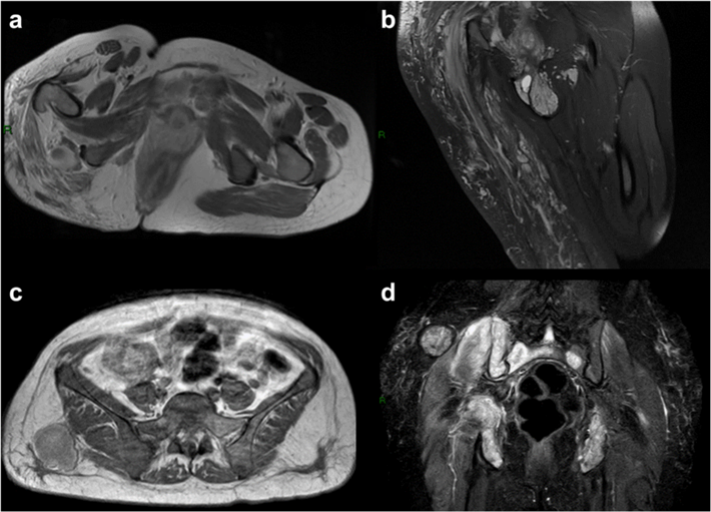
Traditional MR imaging. Axial T1 with contrast media and coronal DP fat of huge angiomatosis of the gluteal region and upper right tight. Either in T1 with contrast or in DP fat weighted image is possible to recognize the huge, mingled, and interspersed, vessels proliferation (a-b). Metastasis of angiosarcoma of the breast involving either the soft tissue of the gluteal region or the bone of sacral and iliac wing (c-d), axial T1 with contrast media and coronal STIR. In T1 only the soft tissue lesion is hyperintense, in STIR both lesion, soft tissue and bone are hyperintense
